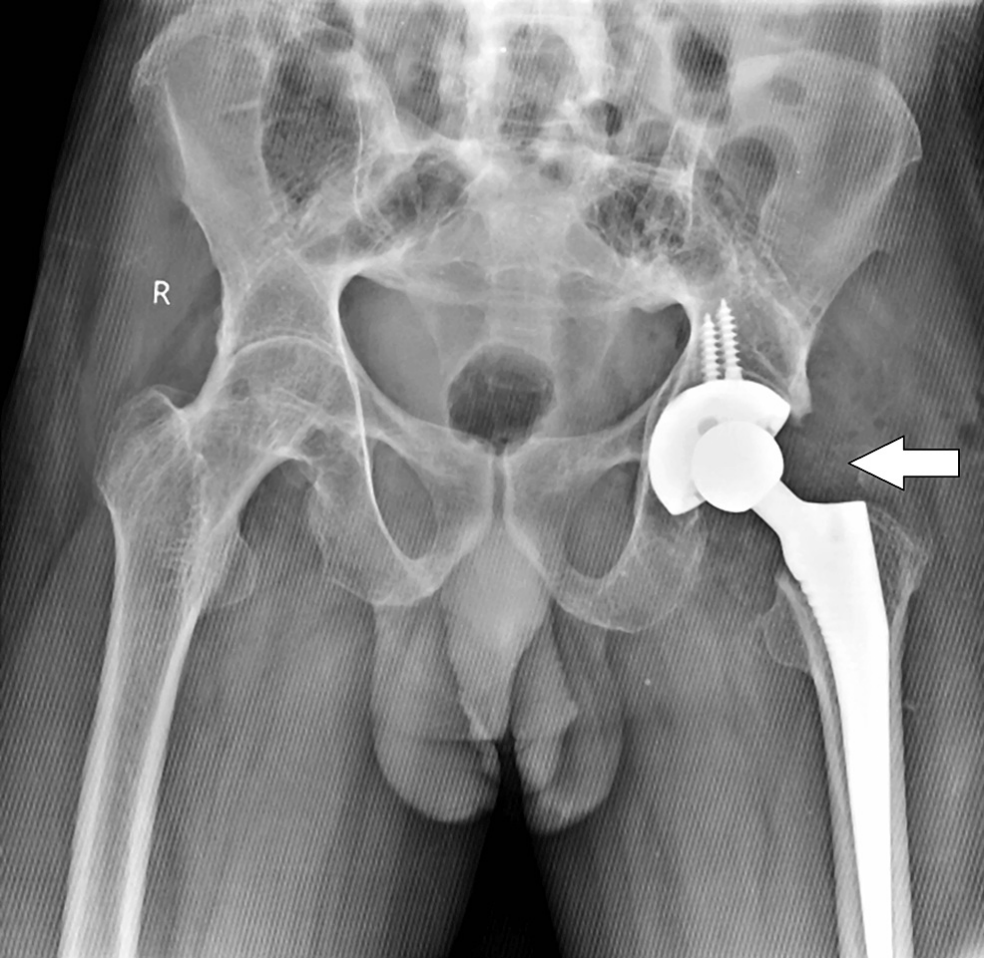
Pelvis X-ray with bilateral hips: postoperative X-ray of the operated case of total hip arthroplasty on the left side with implant in situ
